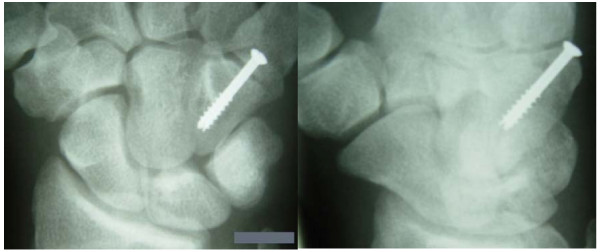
Three month follow up anteroposterior (left) and oblique (right) x-ray views.
